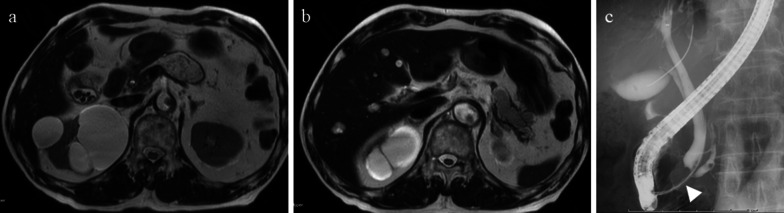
Preoperative imaging findings. a T2-weighted MRI showed a cystic tumor, 48 mm in diameter, in the pancreatic body. b Size of the main pancreatic duct was 18 mm in the pancreatic tail. c ERCP showed that the length of the common channel connecting the bile duct and pancreatic duct was 12 mm (arrowhead)
